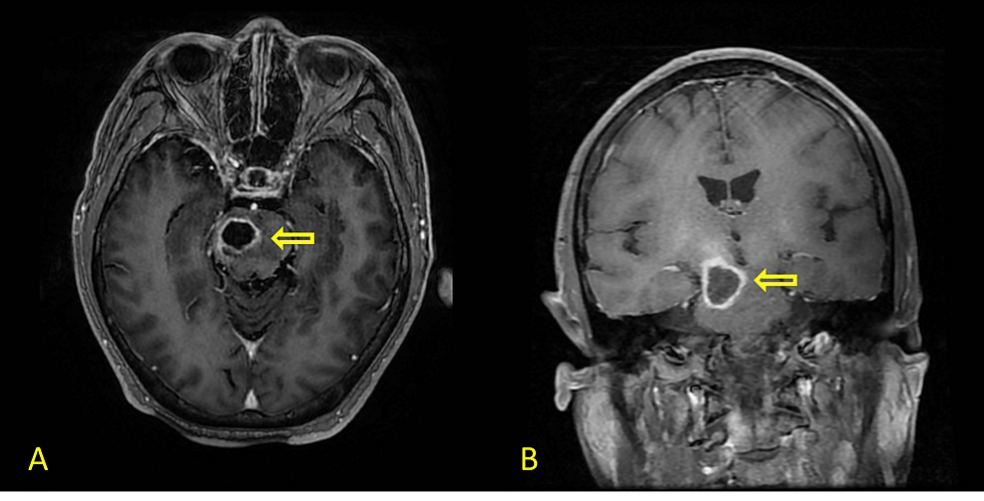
Contrast-enhanced head MRI showing ring-enhancing lesion in right pons (yellow arrow) in both axial (A) and coronal (B) views.
